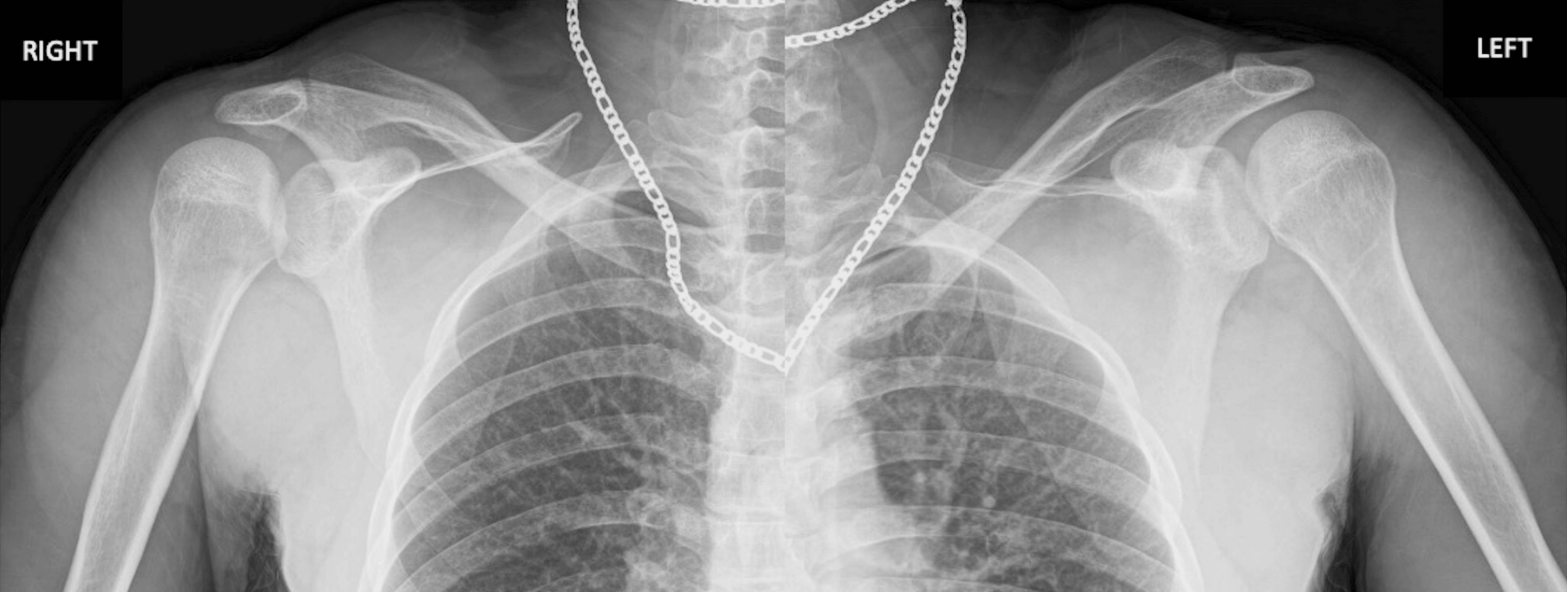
Plain bilateral radiograph of the shoulder showing posterior shoulder dislocation
